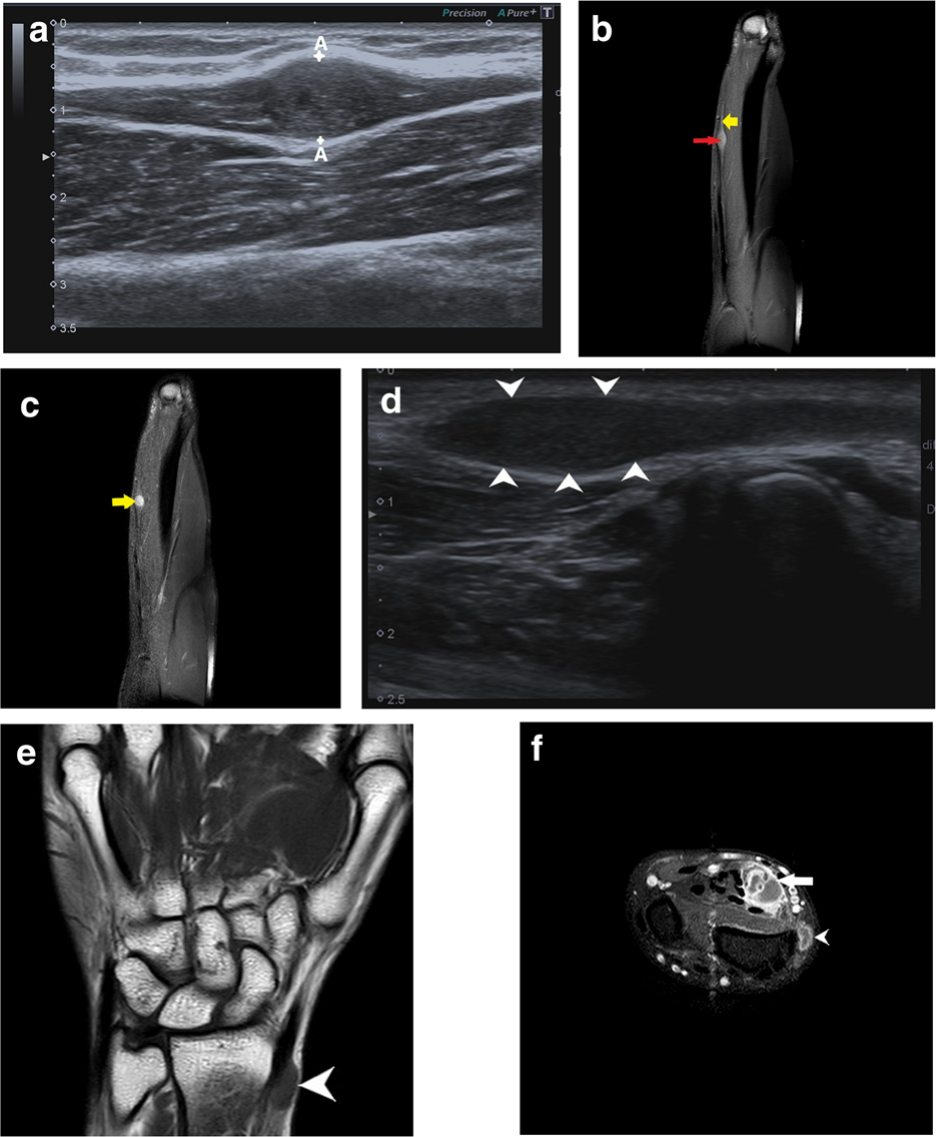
a Characteristic fusiform swelling in continuity of the ulnar nerve can be seen on this ultrasound image, suggestive of peripheral nerve sheath tumour. b PD FS image shows a fusiform swelling which is hyperintense (red arrow) with internal hypointense areas, suggestive of collagenous stroma. The ulnar nerve (yellow arrow) can be seen forming a tail, entering and exiting from the lesion, which was diagnosed to be a PNST. c Post-contrast T1 FS image shows vivid enhancement in the PNST. d Well-defined fusiform hypoechoic lesion (white arrowheads) is noted in continuity of superficial branch of radial nerve in the region of the anatomical snuffbox, just distal to the radial styloid. e Similar lesions are seen on T1-weighted coronal image. A well-defined, T1 hypointense, fusiform lesion (white arrowhead) in continuity with the superficial branch of radial nerve at the distal end of radius can be seen. f Axial post-contrast fat-saturated T1 image showing well-defined hypointense lesion surrounded by an enhancing capsule (arrowhead) in continuity with superficial branch of radial nerve. Another similar lesion (arrow) showing heterogenous contrast enhancement with hypointense areas within can be seen in continuity with the median nerve. This lesion was T2 and PD hyperintense with cystic degenerative changes on ultrasound and MRI. These MR findings fit the description of ancient schwannomas [35]
